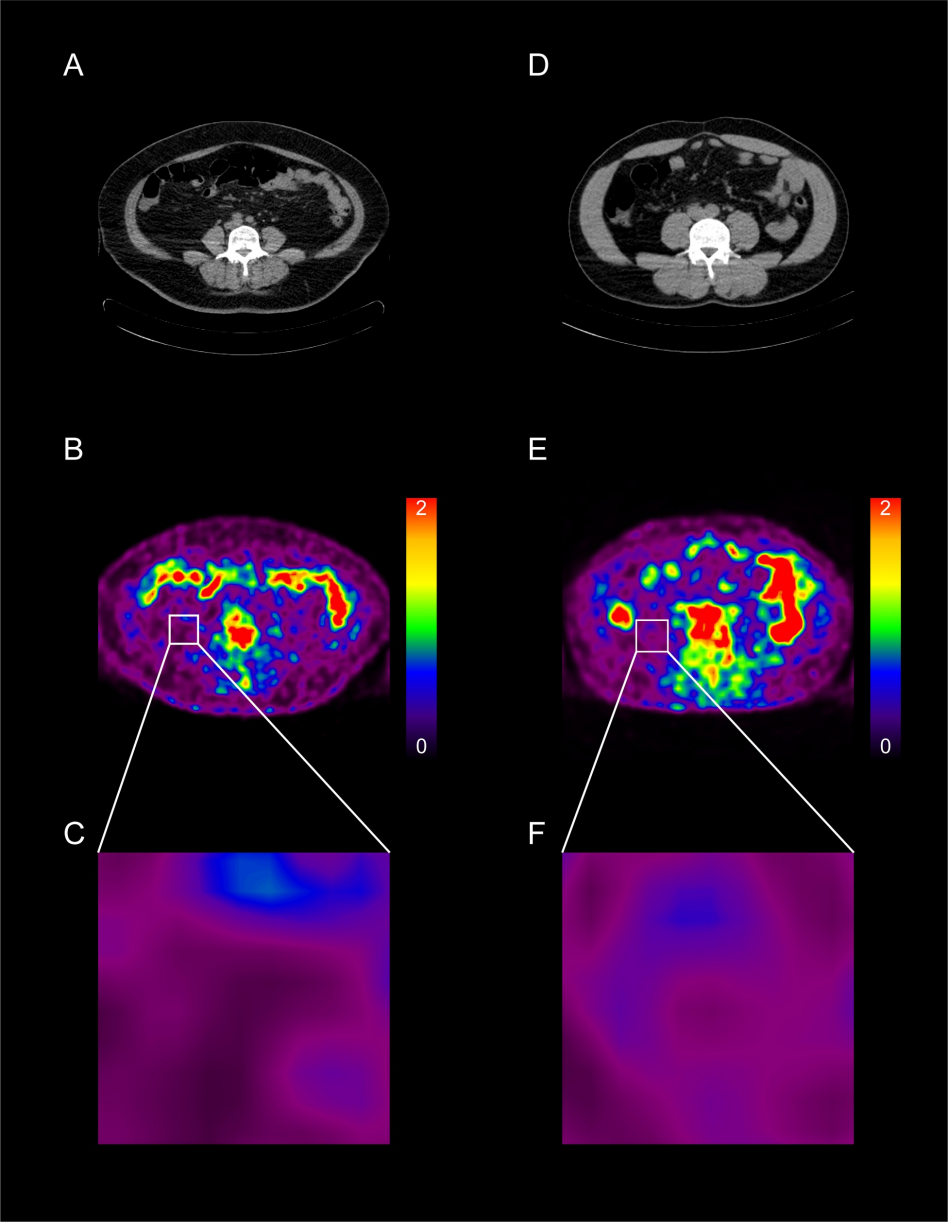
Representative images of ^18^F-FDG PET/CT analysis of VAT and SAT of a MS patient (A-C) and a M(0) patient (D-F). Transaxial CT images at the level of the L4 vertebrae were acquired in both MS and M(0) patients (A and D). Transaxial ^18^F-FDG images corresponding to the above transaxial CT images were acquired (B and E). Magnified ^18^F-FDG images of VAT in both MS and M(0) patients (C and F). SUVs are displayed in a color scale indicating high (red) to low (black) FDG uptake. Different FDG uptake pattern of the VAT region is observed between the MS and the M(0) patients. In the VAT region, MS patient exhibits a large portion of relatively lower FDG uptake area and a small portion of relatively higher FDG uptake area than those of M(0) patient.
